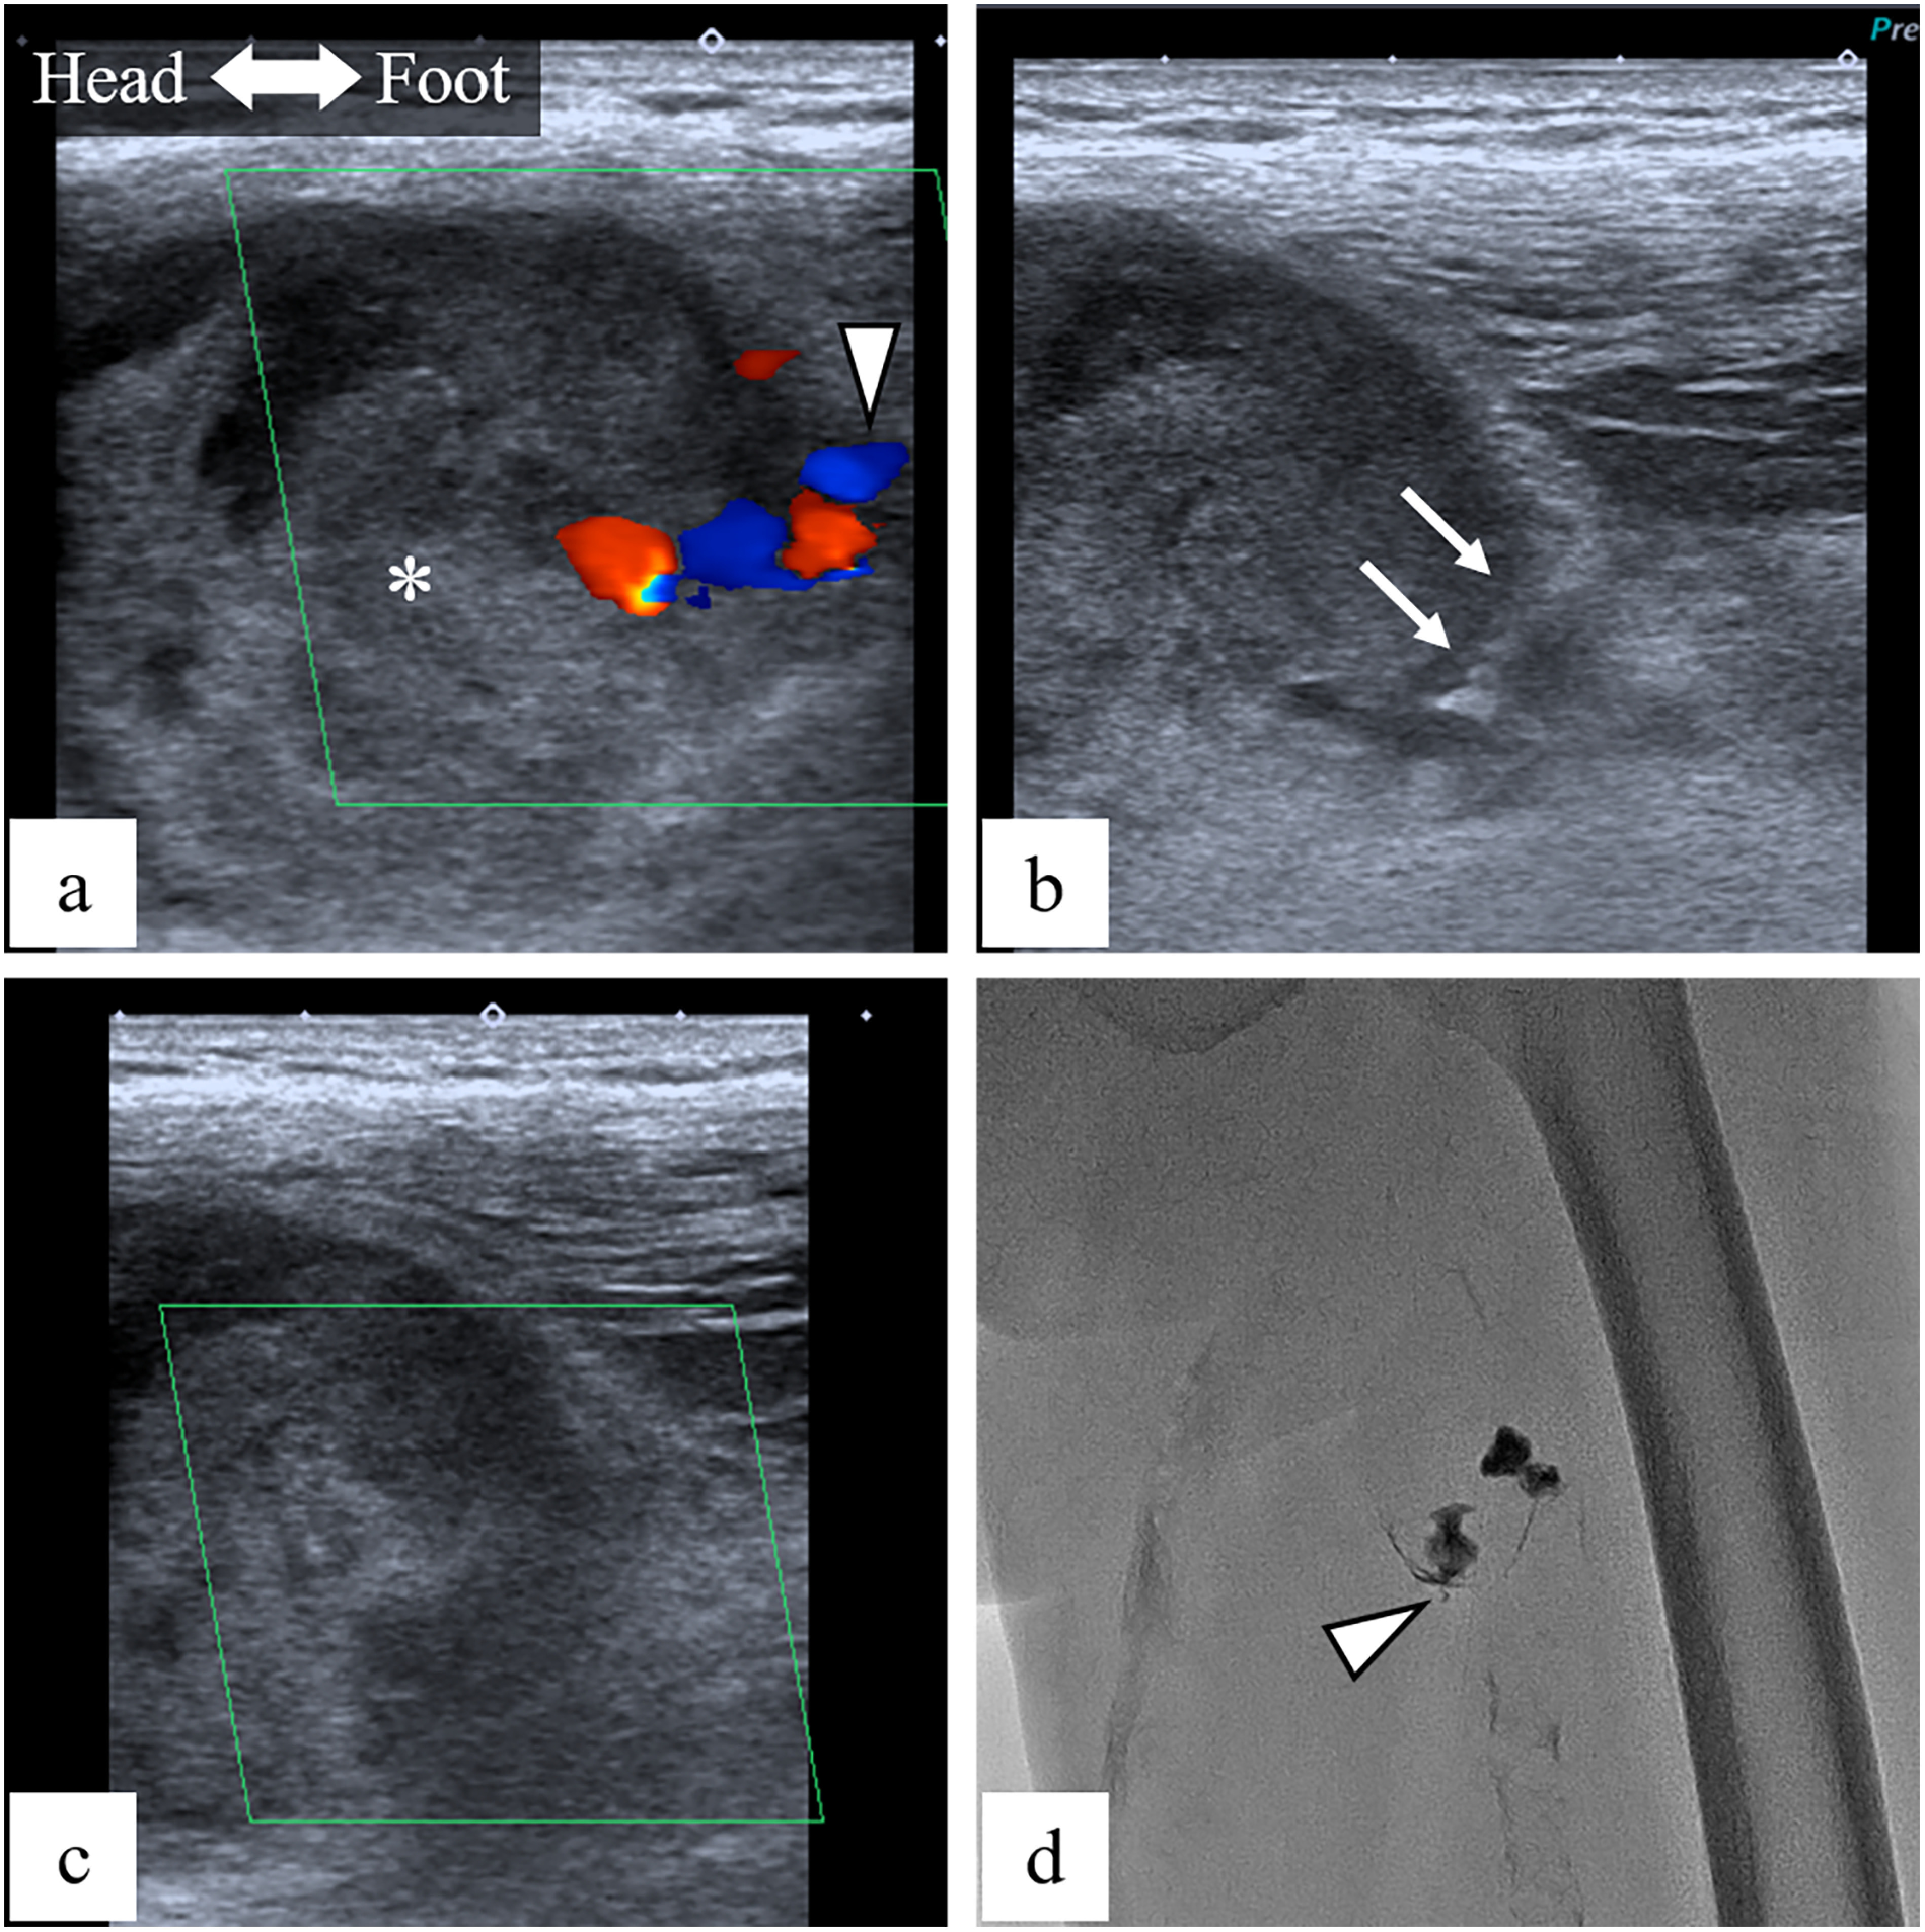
Fig. 3 Direct puncture percutaneous embolization. Color Doppler ultrasonography shows blood flow signals in the distal side of the aneurysm and the proximal portion of the distal artery (arrow) (a). A 21-G needle is introduced into the ostium of the distal artery; arrows indicate the puncture needle line (b). Color Doppler ultrasonography after the embolization shows that the blood signals have completely disappeared (c). The X-ray image after embolization shows deposition of Lipiodol in the distal side of the aneurysm and the proximal portion of the distal artery (arrowhead) (d).
